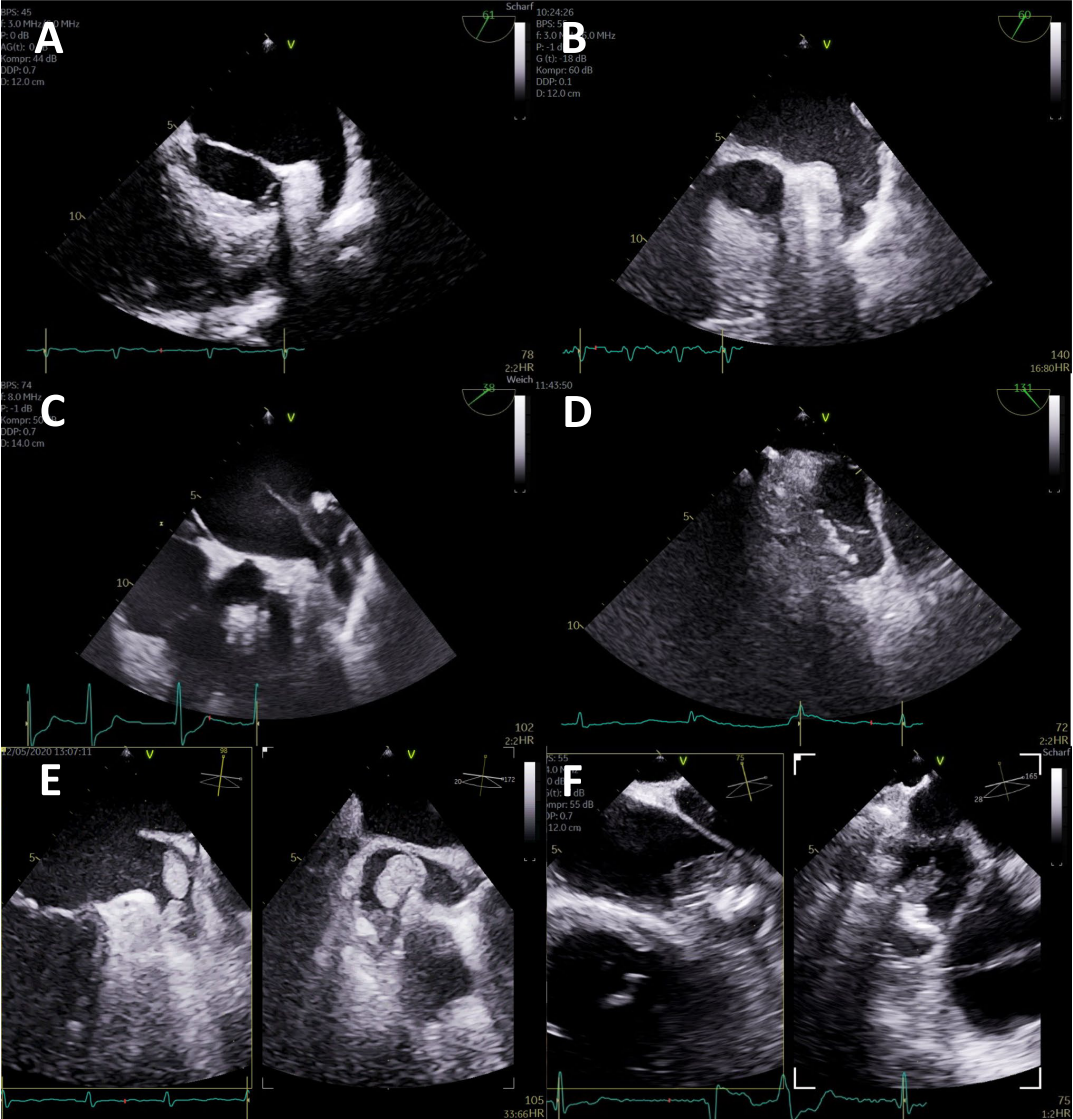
Echocardiographic analysis of left atrial appendage (LAA) for thrombus detection. A Oblique—chamber view of LAA with normal LAA; B corresponding view with low degree of spontaneous echo contrast (SEC); C corresponding view with progressive degree of SEC shown by the formation of streaks; D corresponding view with severe degree of SEC shown by the formation of powder folding in the LAA apex; E biplane views of large thrombus formation in the LAA; F biplane views of large thrombus formation on a LAA closure device
